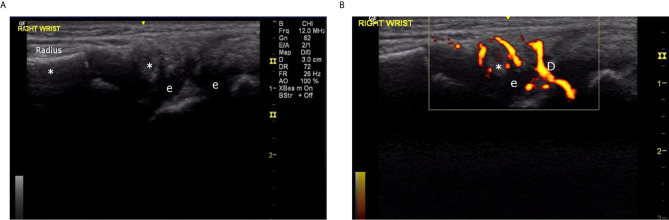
(A) Right wrist, 4^th^ compartment, longitudinal view, greyscale (6 year old male, Case 2), demonstrating proliferative synovitis (*) and effusion (e). (B) Right wrist, 4^th^ compartment, longitudinal view, color Doppler (6 year old male, Case 2), demonstrating intense 3+ Doppler signal (D) in proliferative synovium.
